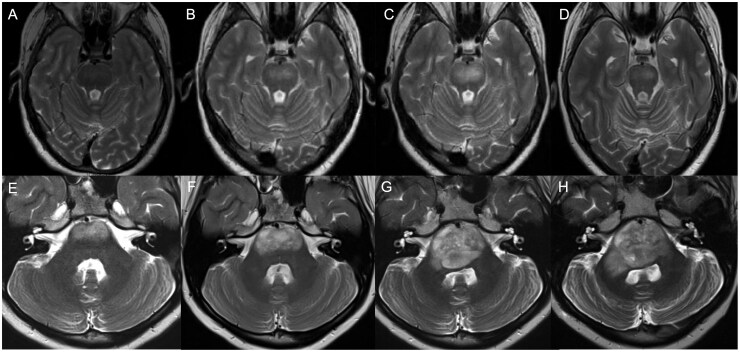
(A-D) Magnetic Resonance Imaging of Case 2 (15.8-year-old patient, 10 years after adjuvant RT for CP). Serial axial T2-weighted images one year earlier (A), and at the first time a hyperintense ill-defined lesion centered in the basis pontis was identified on follow-up imaging (B). Eight months later, the pontine lesion had increased in size, reaching 2/3 of the cross-sectional area of the basis pontis, although remaining relatively homogenous (C), therefore leading to a biopsy that enabled to reach the diagnosis of RIG. At that time the patient was asymptomatic. MRI performed after re-irradiation shows a decrease in size and intensity of the basis pontine lesion due to favorable response to RT (D). (E-H) Magnetic Resonance Imaging of Case 3 (16-year-old patient, 3 years after adjuvant RT for locally recurrent CP). Serial axial T2-weighted images during surveillance of the asymptomatic patient. Initial faint hyperintense lesion within the basis pontis (E). One year later, the ill-defined T2-hyperintense lesion of the basis pontis had increased in size with subtle ventral growth within the prepontine cisterna (F). One year and a six months later, the lesion became increasingly heterogeneous, occupying the entire surface of the basis pontis with ventral exophytic growth engulfing the basilary artery, these findings highly suggestive of DIPG despite the absence of symptoms (G). Two months later after the radiologic diagnosis, 1 year and 8 months after the pontine lesion had appeared, the mass showed further extension into the right middle cerebellar peduncle, coinciding with the onset of symptoms and prompting biopsy (H).
